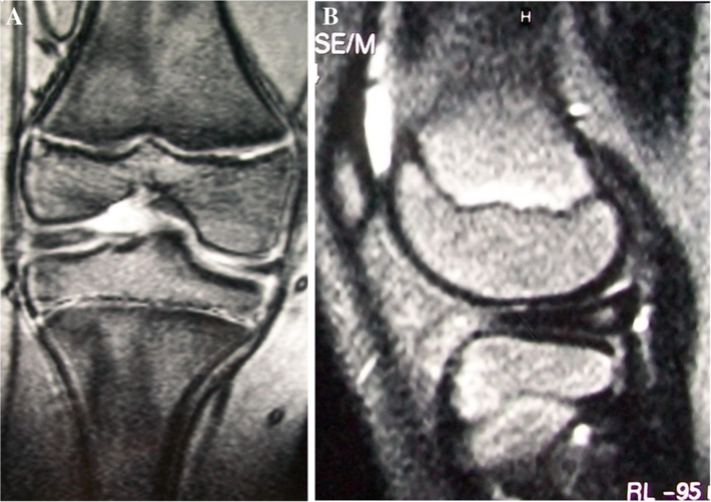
Coronal (A) and sagittal (B) MRI views of the right knee performed when the patient was 11 year-old showing an incomplete discoid lateral meniscus.
